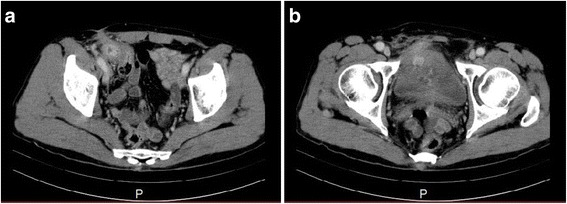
Abdominal computed tomography findings. a A poorly defined mass is observed to be in contact with the cecum. b The mass is also in contact with the bladder wall
